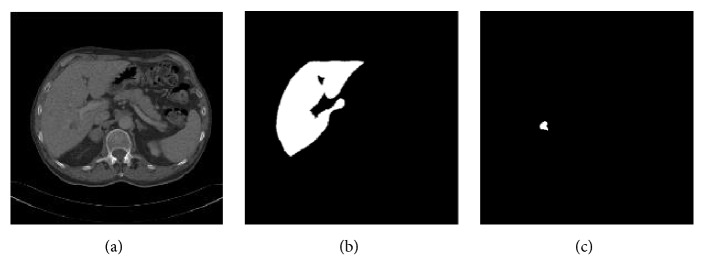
An example of the CT image and its labels (liver and tumor). (a) An abdominal CT image. (b) The liver label. (c) The tumor label.
